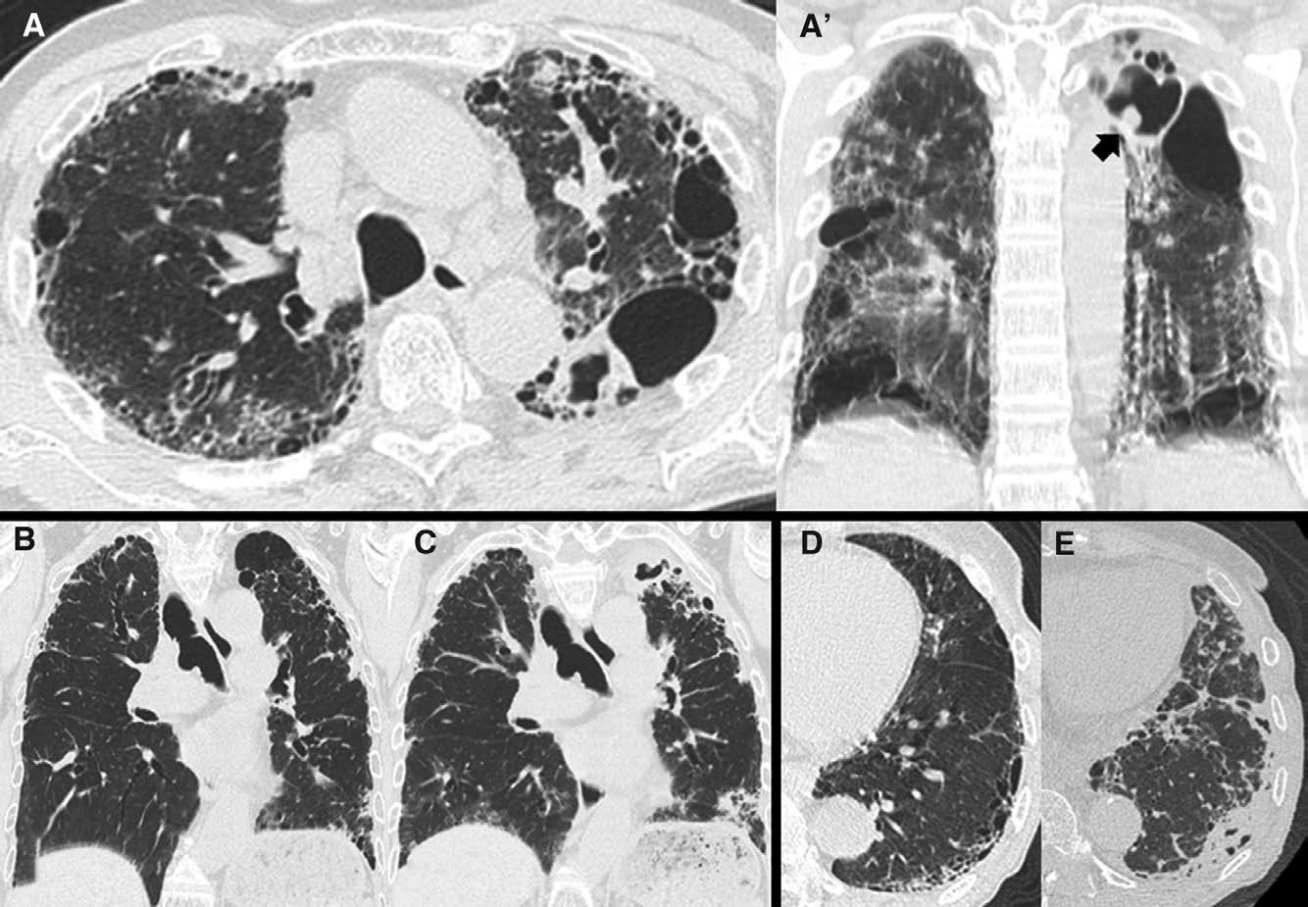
Chest CT images of typical cases of CPA. (A/A′) Chest CT scan of a 77-year-old man with emphysema with interstitial lung disease showed a fungus ball (black arrow) in the cavitary lesion with consolidation in the left upper lobe. (B/C) Chest CT scan of a 71-year-old man revealed fibrosing interstitial lung disease as pleuroparenchymal fibroelastosis in (B). In the same patient 4 months after initiation of corticosteroid therapy (C), pericystic infiltartion and pleural thickening in the left upper lobe of the CT image were apparent, and he was subsequenlty diagnosed as having CPA. (D/E) Chest CT scan of a 72-year-old woman revealed reticulation with traction bronchiectasis in the subpleural lung as probable UIP pattern (D). In the same patient 2 years and 2 months later (E), localized consolidation was apparent in the subpleural fibrotic lesion, and she was diagnosed as having CPA. CPA = chronic pulmonary aspergillosis, CT = computed tomography.
